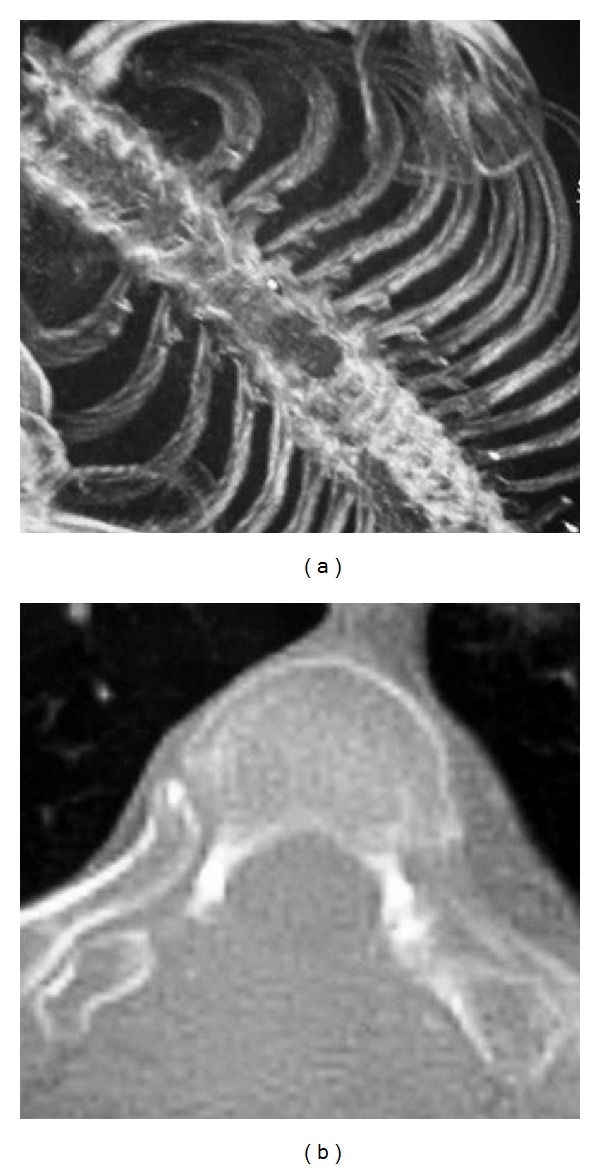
Postoperative (1 year) CT scan of the lesion at Th3-Th4 level, demonstrating laminectomy and absence of local recurrence.
